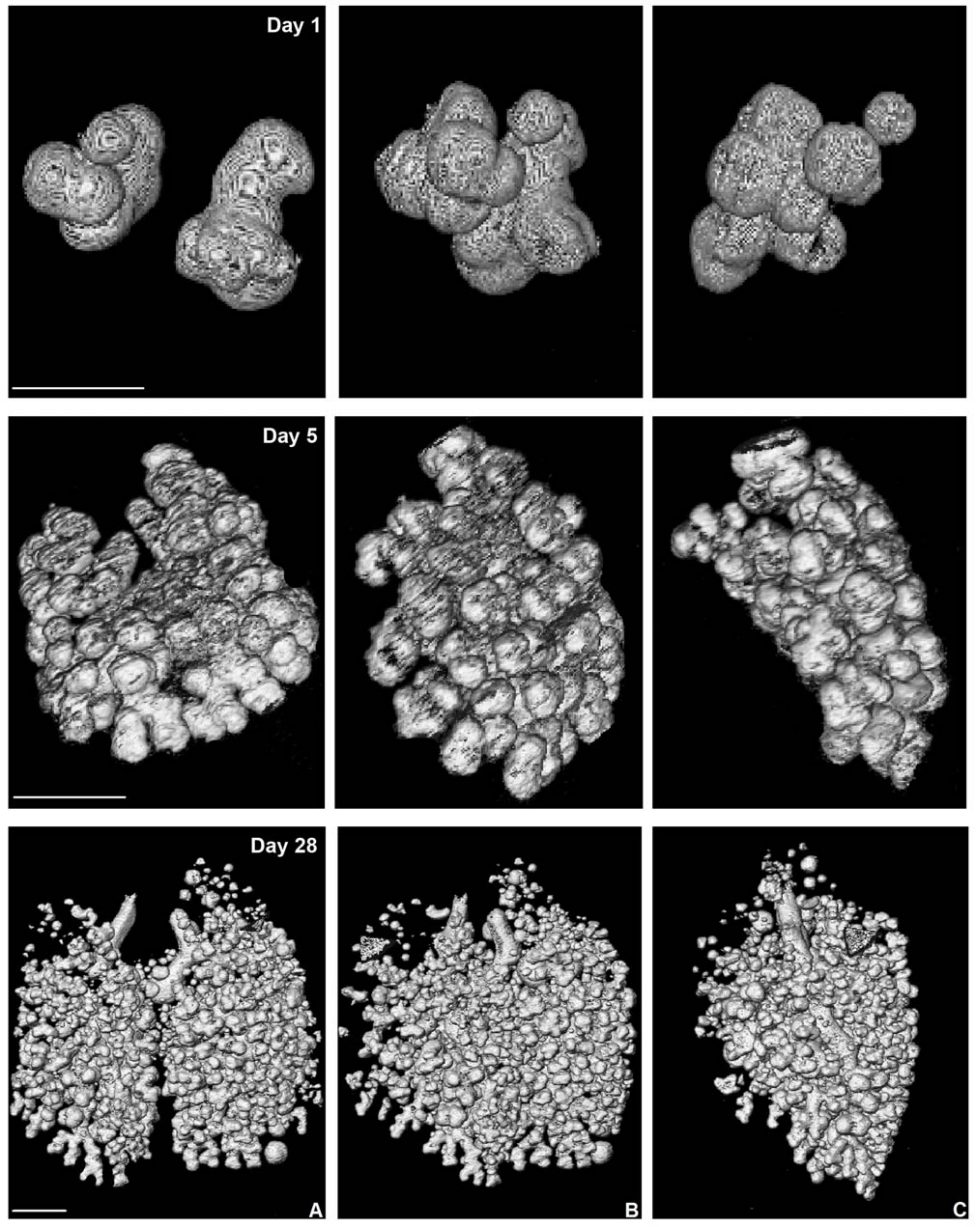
3-Dimensional volume rendering from fat-tailed dunnart computed tomography data sets. Shown are three frames captured over 90 degrees of lung rotation. The series consists of an anterior view (A), a lateral view (C) and the mid-point between them (B), further demonstrating the increase in air sac number, and changes to air sac shape throughout the first weeks of lung development in the fat-tailed dunnart. Scale bar represents 200 µm.
